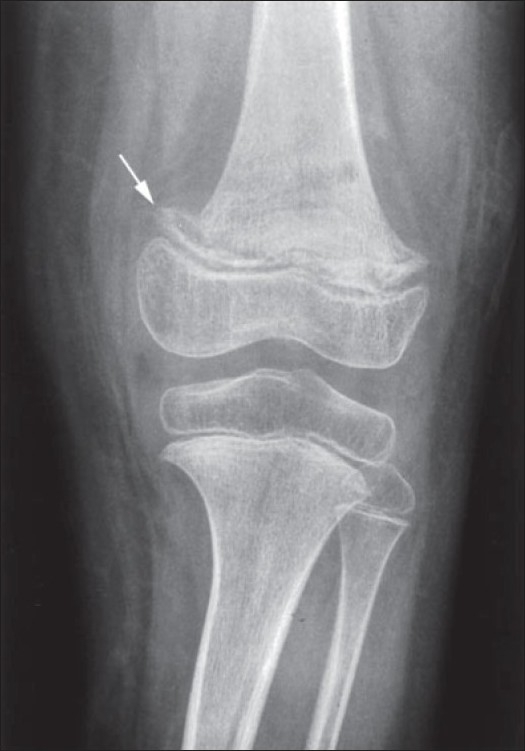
X-ray of the lower and upper limbs (arrow indicates scurvy line).
